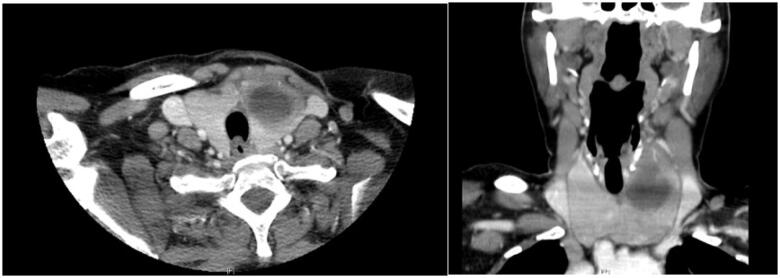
Neck computed tomography scan showing a hypodense nodule of thyroid left lobule measuring 30 × 35 × 35 mm, with tracheal compression.
